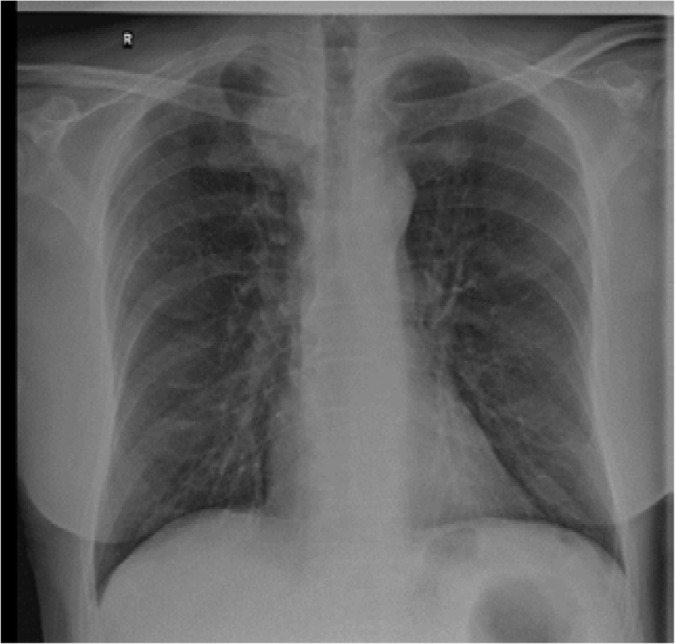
Chest X-ray showing round mass in upper right lobe.
